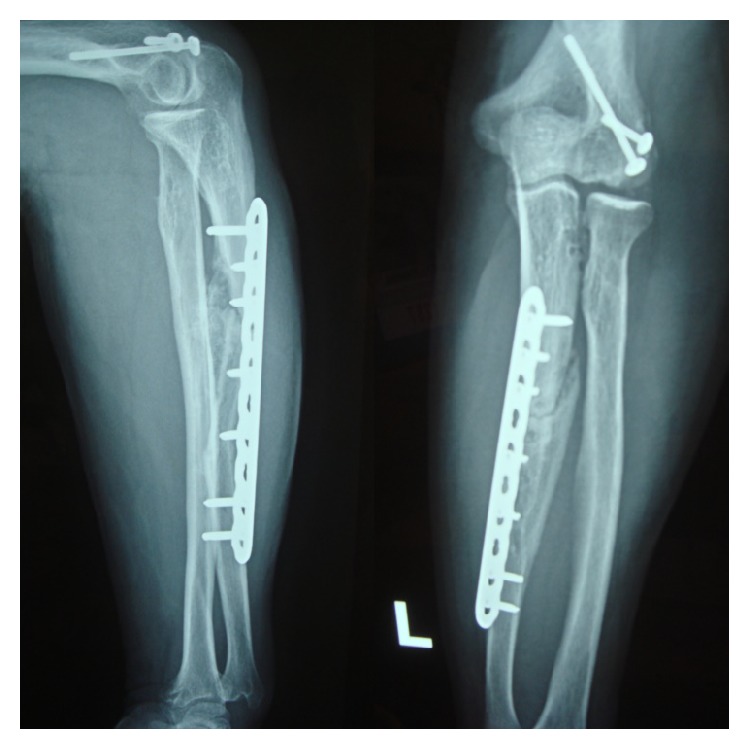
3-month follow-up X-ray revealing adequate healing.
